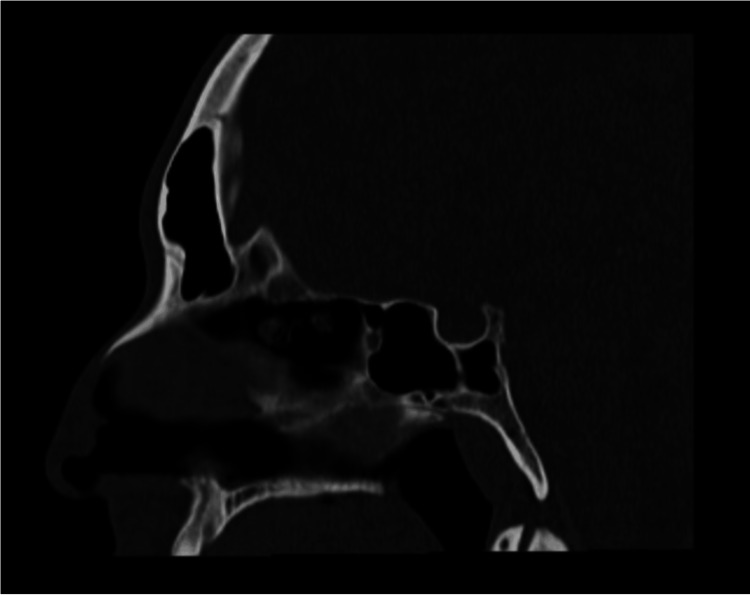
CT scan showing the absence of abnormalities and tumors in the nasolacrimal system. CT, computed tomography.
